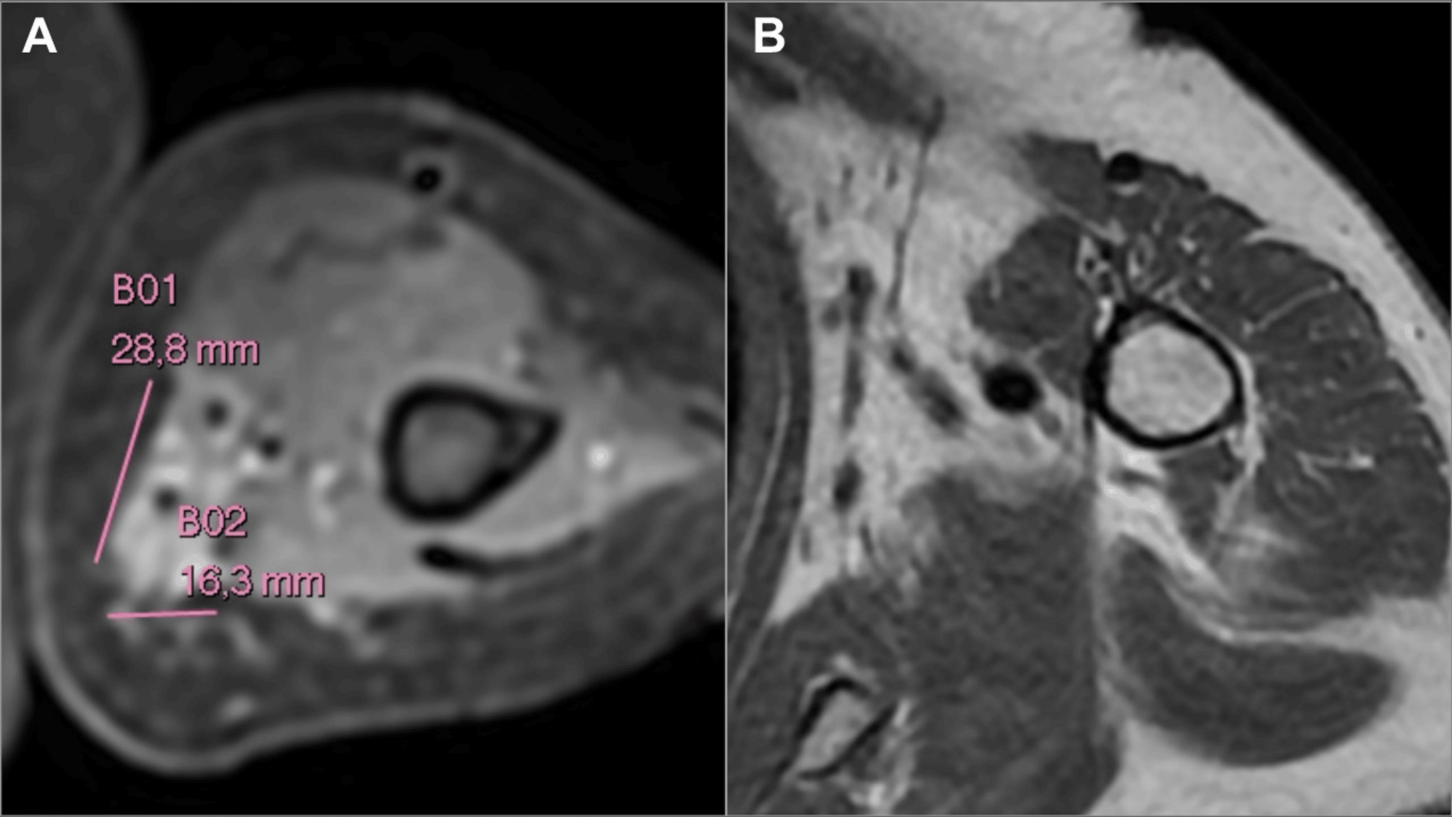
After-treatment MRI MRI after completing six cycles of nivolumab shows a reduction in the size of the lesion in the left arm and no signs of axillary lymphadenopathy. (A) T2 STIR sequence. (D) T1 sequence.
